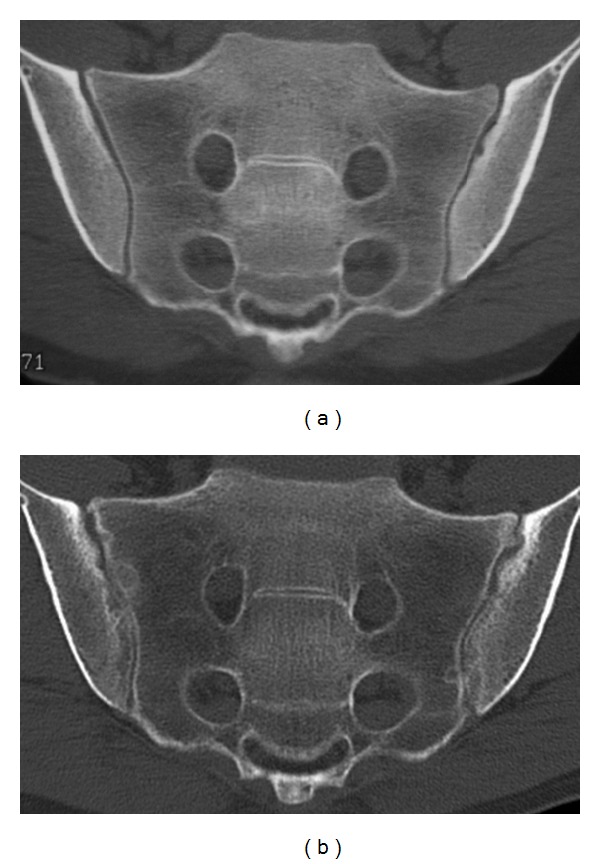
A case with changes from unilateral to bilateral sacroiliitis in a 25-year-old male with 1–3 years history of suspected spondyloarthropathy. (a) The initial CT shows unilateral sacroiliitis with subtle erosions in the left sacroiliac joint on the iliac side, with surrounding mild inflammatory sclerosis. On the right, there is poor definition of the subchondral cortical bone. (b) Seven years later, there is progression to definite bilateral sacroiliitis.
